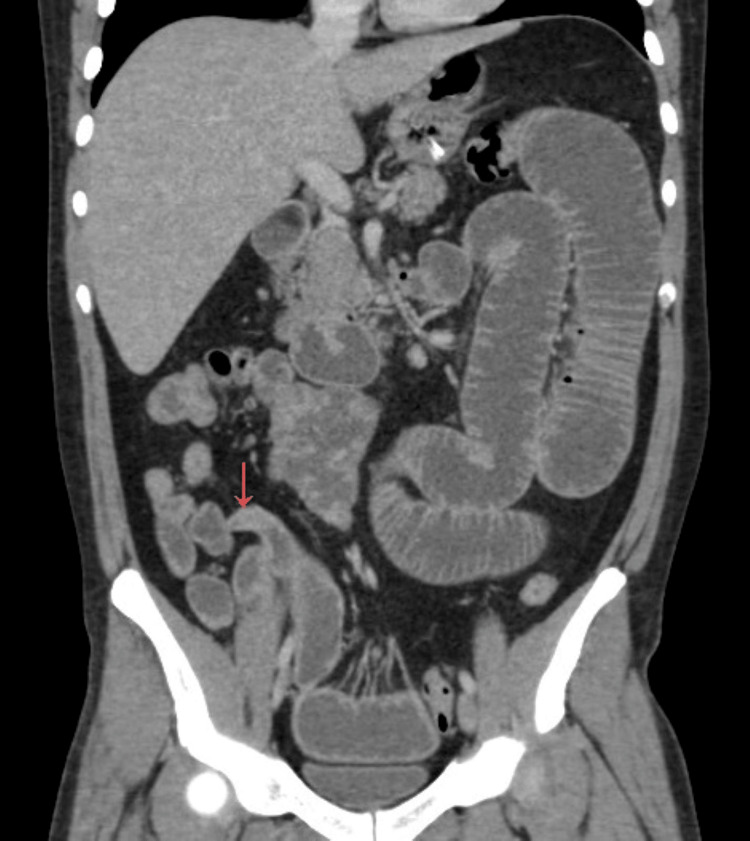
Coronal contrast-enhanced CT reconstruction of Meckel’s diverticulum. Coronal contrast-enhanced CT image demonstrating a blind-ending diverticular structure arising from the antimesenteric border of the distal ileum (arrow), consistent with Meckel’s diverticulum, with improved visualization of its anatomical orientation.
